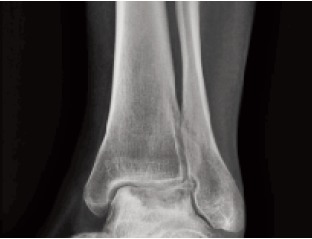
Fig. 2: Showing posteromedial necrosis on MRI of the talus. F2:
